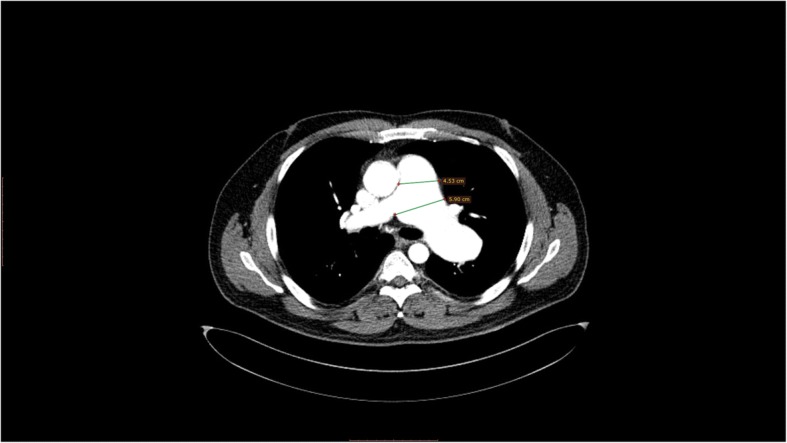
Chest computed tomography angiogram scan (CT) showing: Dilated pulmonary trunk (4.5 cm), Left main pulmonary artery (6 cm), Right main pulmonary artery (2.3 cm)
